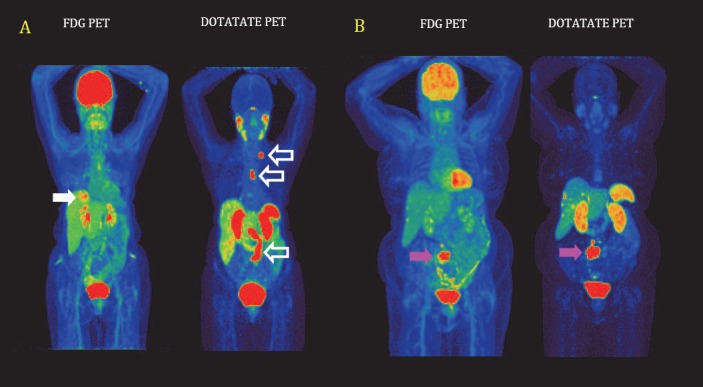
The images show two patients who underwent contemporaneous [F-18]-FDG and [Ga-68]-DOTATATE imaging. (A) This is a 72 year old female patient diagnosed with carcinoid and known metastatic disease in the liver and abdominal lymph nodes. The liver lesion (closed white arrow) was identified on FDG imaging alone. Lymph nodes (open white arrows) with increased focal uptake were only demonstrated on DOTATATE imaging. FDG positive / DOTATATE negative lesions are more likely to be poorly differentiated and higher grade whereas DOTATATE positive / FDG negative are usually well differentiated and lower grade. (B) A 73 year old female patient with metastatic small bowel carcinoid, currently being treated with [Lu-177]-DOTATATE therapy. The known small bowel lesion can be seen on both the FDG and DOTATATE images (pink arrows). Used in combination, FDG and DOTATATE imaging allows the degree of differentiation of the lesions to be assessed which is a guide to the most appropriate form of therapy (Peptide Receptor Radionuclide Therapy (PRRT) or conventional chemotherapy). All patient scans were performed in the Department of Nuclear Medicine, Royal North Shore Hospital, Sydney using a Siemens Biograph mCT Time-of-Flight PET scanner (*Biograph mCT.s/x 64, Siemens Healthcare, IL, USA*)
